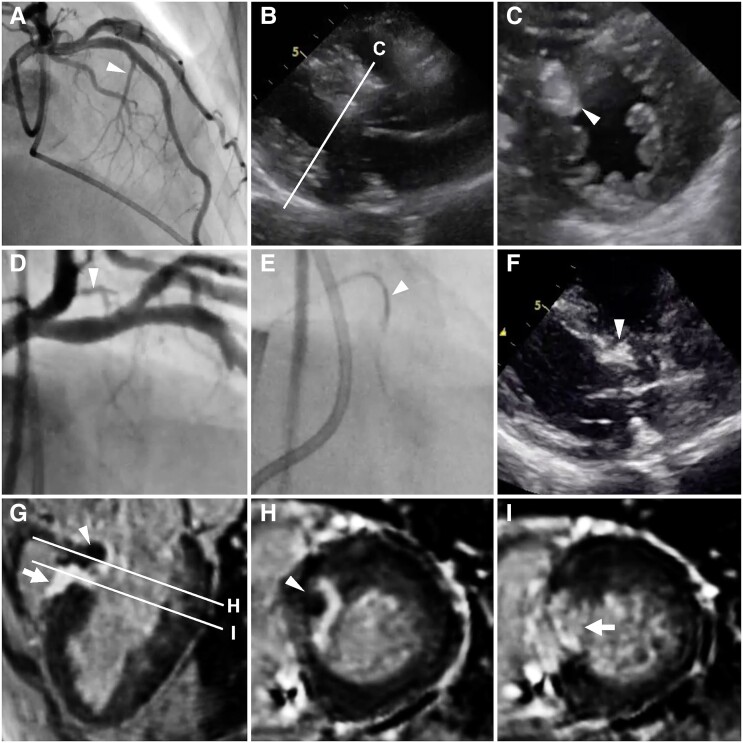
A 65-year-old female patient with obstructive hypertrophic cardiomyopathy was referred to our hospital for septal reduction therapy. Echocardiography revealed left ventricular outflow tract obstruction (LVOTO) with systolic anterior motion of the mitral leaflet and no abnormal structure related to the obstruction. Coronary angiography identified the major septal branch originating from the left anterior descending artery to be a target branch for alcohol septal ablation (ASA) (*Panel A*). In the initial procedure, under septal branch occlusion by a 2 mm over-the-wire balloon (see *Movie S1*), the culprit myocardial bulge was ablated with 2 mL of ethanol (*Panels B* and *C*), with the resting gradient reduced from 100 to 3 mmHg and peak serum creatine kinase level of 1330 U/L. One year later, exertional dyspnoea recurred with LVOTO at the most-basal septum. In coronary angiography, a sub-branch from the left circumflex artery was deemed to be a target vessel for ASA (*Panel D*). In the repeat procedure, we used a Corsair Pro microcatheter [tip: 1.3 Fr (0.42 mm), shaft: 2.6 Fr (0.87 mm), Asahi Intec, Nagoya, Japan]. A tip-injection into the microcatheter wedged on the sub-branch confirmed the perfusion to the culprit basal septum and no ethanol leakage to any other vessel (*Panel E*, *Movie S2*). Injection of 1.2 mL of ethanol resulted in ablation of the residual myocardial bulge (*Panel F*). The gradient reduced from 42 to 2 mmHg at rest and 87 to 6 mmHg on Valsalva with peak serum creatine kinase level of 453 U/L. Cardiac magnetic resonance imaging proved the additional ablation of the most-basal septum (*Panels G* and *H*, arrowheads) next to the scar of the initial ASA (*Panels G* and *I*, arrows). No relapse of LVOTO was observed for three-year follow-up.
